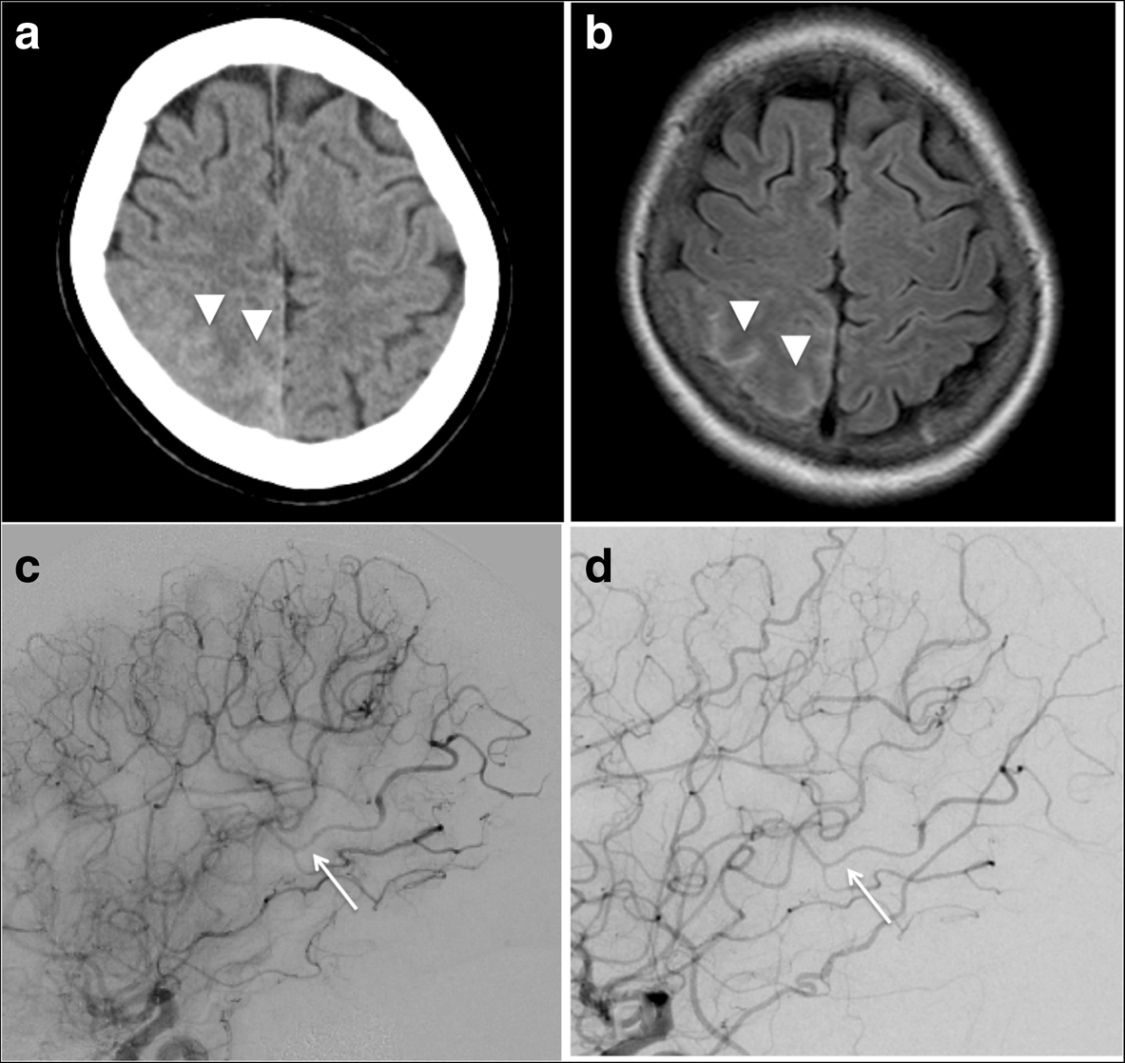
Neuroimaging findings of Case 2. a CT scan of the head shows a subtle cortical subarachnoid hemorrhage along the right parietal sulci (*arrow heads*). b FLAIR sequence of the MRI shows the distribution of a subarachnoid hemorrhage (*arrow heads*). c Lateral magnified view of the right internal carotid angiogram, late arterial phase, shows a stenosis (*arrow*) and post-stenotic delayed opacification in the area of peripheral middle cerebral artery. d Lateral magnified view of the right internal carotid angiogram obtained one week after onset shows a disappearance of stenotic findings and normal perfusion (*arrow*)
